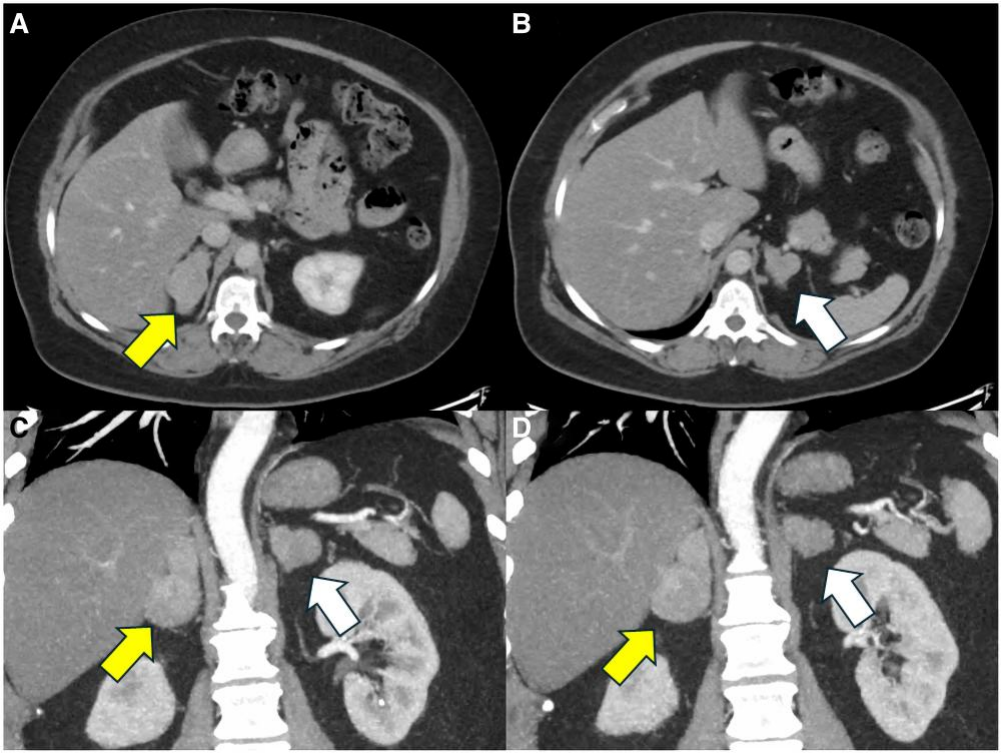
Representative CT scan images from the patient with MACS in the setting of CAH. CT scan of the abdomen showing bilateral adrenal lesions, with the right (yellow arrow) and left (white arrow) adrenal lesions, on axial (A and B) and coronal (C and D) views. All CT scan images depicted are in venous phase (60 seconds following contrast injection) according to a modified adrenal protocol of the Carling Adrenal Center and Hospital for Endocrine Surgery. The dominant right adrenal mass (5.1 cm, 21.5 Hounsfield units) and a left mass (2.7 cm, 11.5 Hounsfield units), indicating adenomas contributing to the recent development of MACS. Abbreviations: CAH, congenital adrenal hyperplasia; CT, computed tomography; MACS, mild autonomous cortisol secretion.
